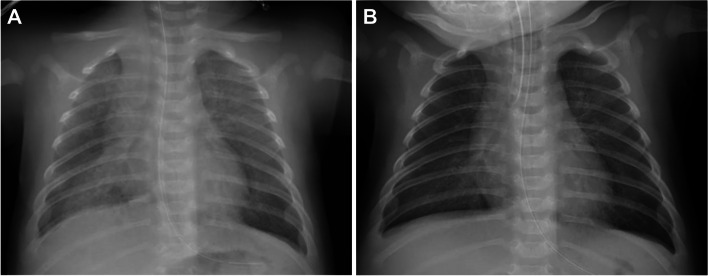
A Chest radiograph obtained in the operating room 15 min after extubation, showing bilateral diffuse pulmonary infiltration. B Chest radiograph 9 min after re-intubation, showing an improvement in diffuse pulmonary infiltration
